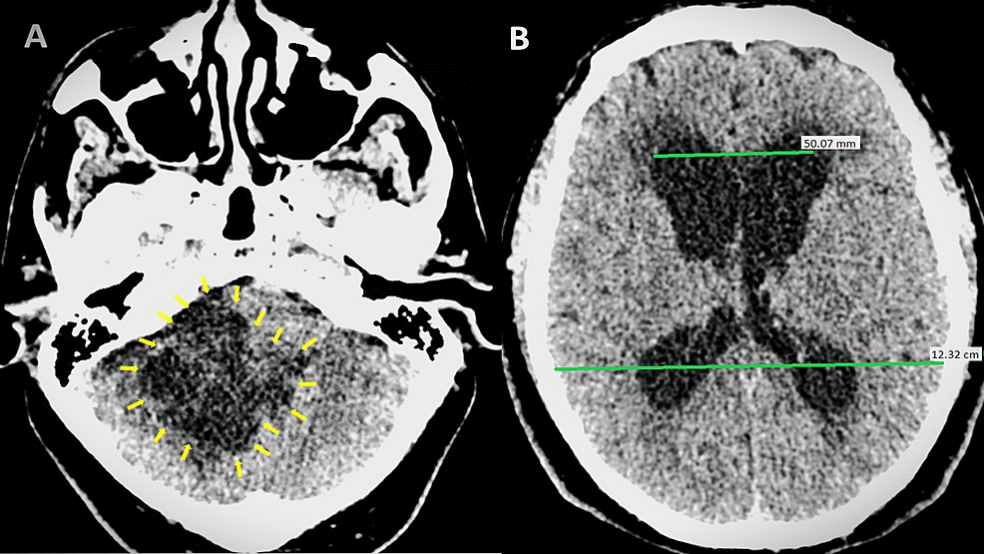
Non-contrast CT scan. (A) Axial scan at the level of the posterior fossa showing a multilobulated hypodense mass (yellow arrows). (B) A supratentorial compartment in which acute hydrocephalus was observed with an Evans index of 0.4 and transependymal edema.
